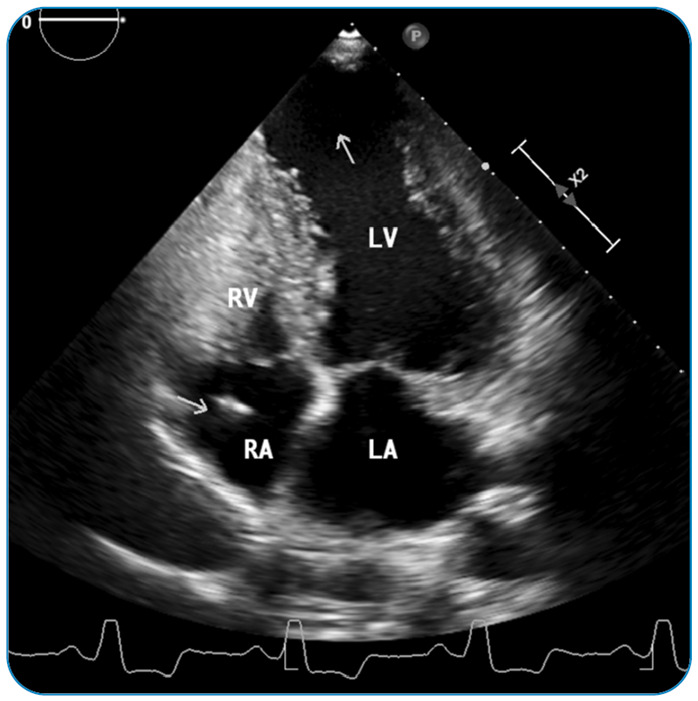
Echocardiographic image at the apical four-chamber view of a patient with Chagas disease presented with stroke. A large left ventricular apical aneurysm (white arrow) is seen. The right ventricle has a normal size with a pacemaker wire in the right atrium (arrow). RV: right ventricle, LV: left ventricle, RA: right atrium, LA: left atrium.
